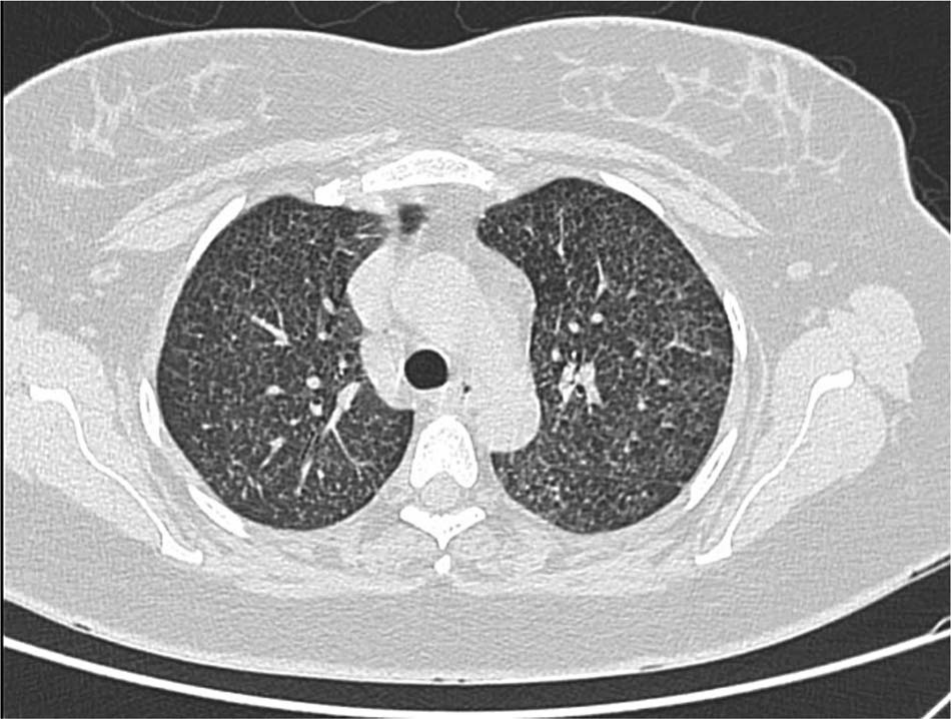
HRCT showing centrilobular nodules and ‘tree-in-bud’ opacities.
